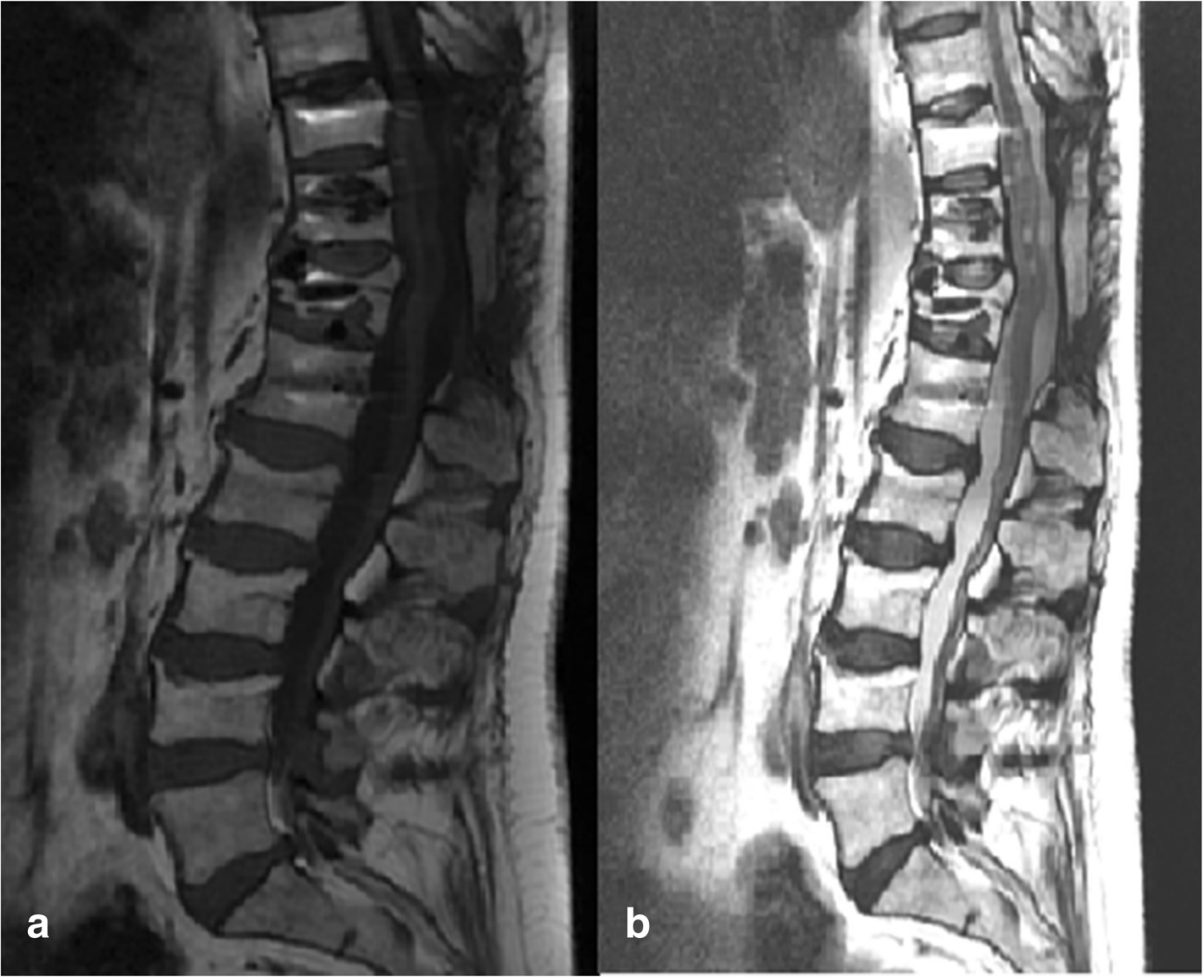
Magnetic resonance imaging 8 weeks after oral prednisolone and epidural administration of methylprednisolone acetate showing no regrowth of the mass. a T1 sagittal view. b T2 sagittal view
